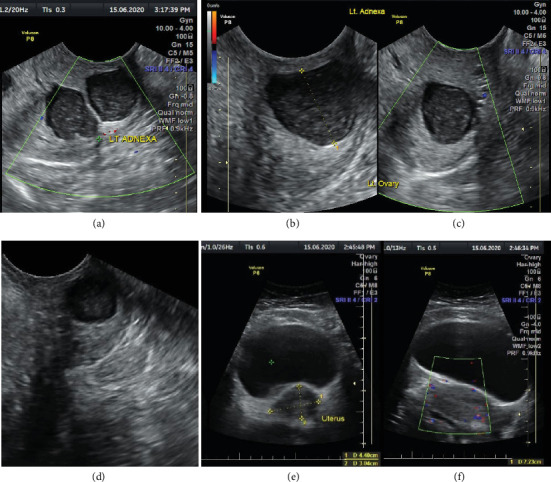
Pelvic ultrasound images (a–c) (left adnexa) and (d) (right adnexa): demonstrating multiple rounded and ovoid hypoechoic lesions some with septa. The largest lesion measured 1.52 × 1.5 cm (b). Lesions show homogeneous low-level internal echoes with posterior acoustic enhancement. No colour flow within lesions upon colour Doppler application, features suggestive of chocolate cysts. (e, f) The uterus in transverse and longitudinal views, with normal size, echogenicity, and normal myometrial vascularity.
